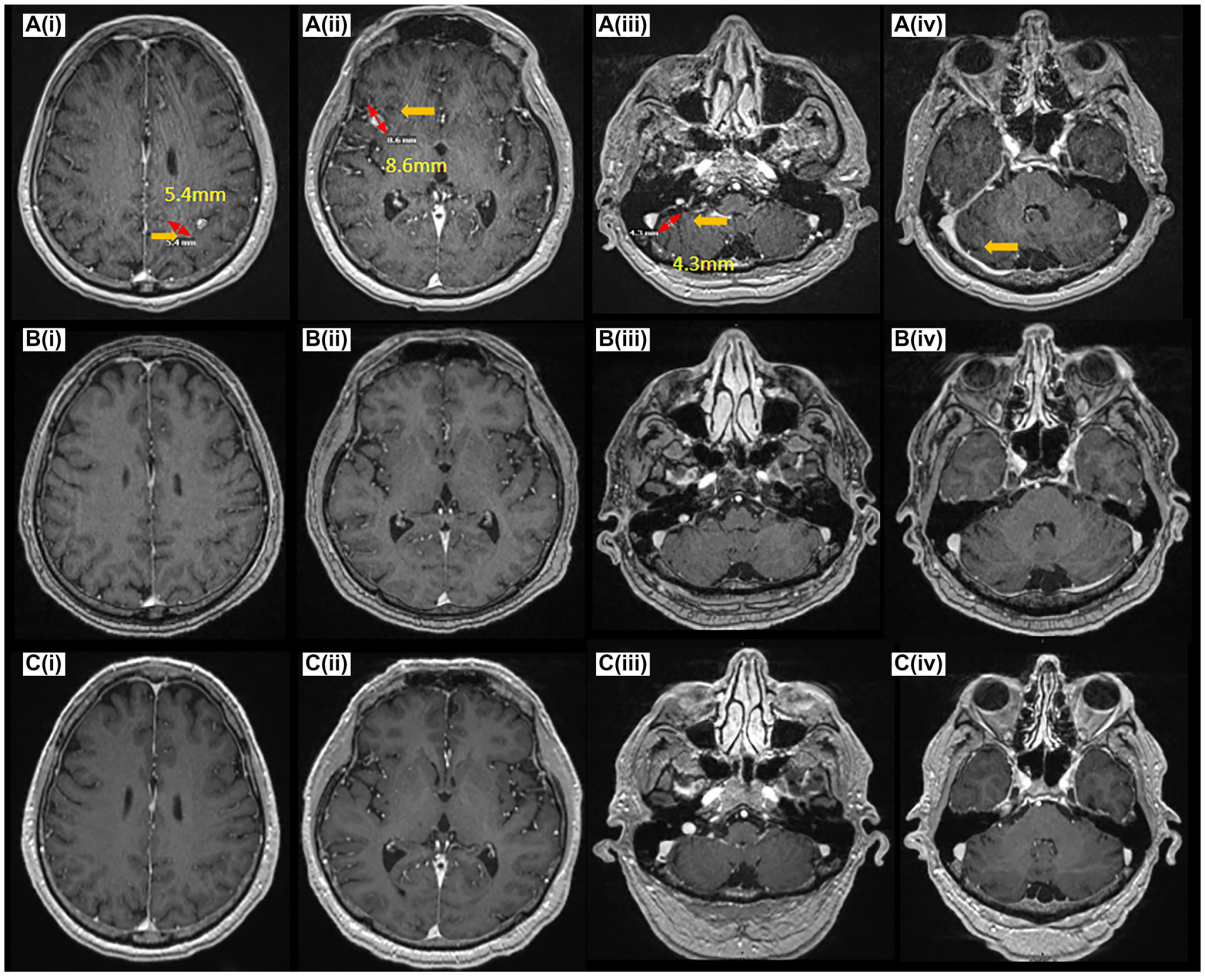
CNS disease response on amivantamab. MRI Brain at the time of pre-treatment (A(i–iv)), 6 months (B(i–iv)), 19 months (C(i–iv)) post-treatment demonstrating complete resolution of CNS disease at 6 months (B(i–iv)), and 19 months (C(i–iv)).
